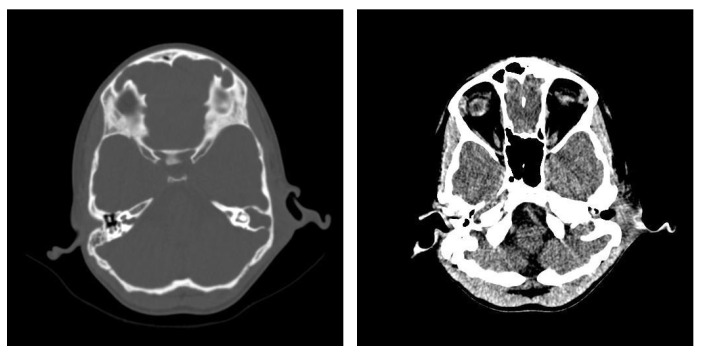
Patient with the diagnosis of acute suppurative polypous exteriorized retroauricular acute polypoid otomastoiditis. CT examination in axial, bony window, and parenchymal sections revealed underdeveloped mastoid air spaces on the left side, tissue formation in the external auditory canal, and diffuse infiltration of the retroauricular soft tissues.
